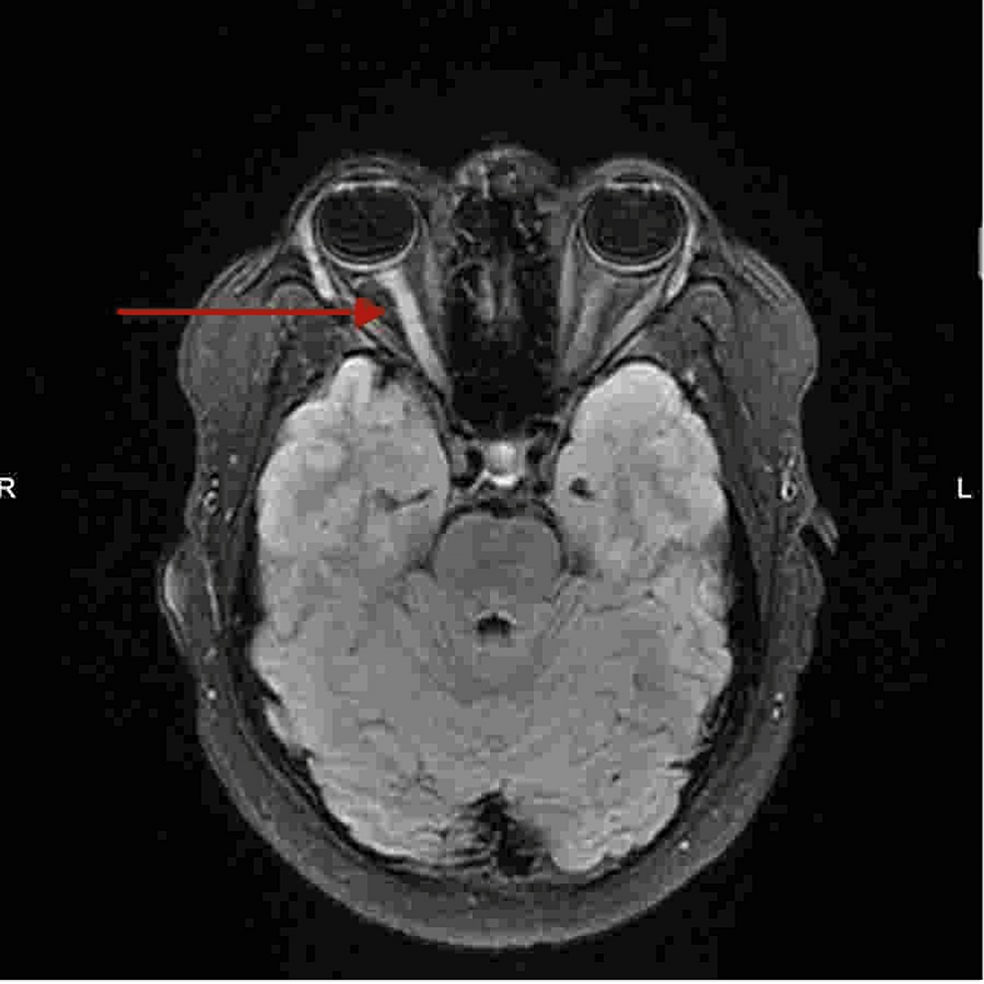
MRI of the brain and orbit T2/FLAIR signal of the aforementioned segments of the right optic nerve is also increased compared to the left side with increased contrast enhancement more prominent at the pre-chiasmatic segment (arrow). MRI: magnetic resonance imaging, FLAIR: fluid-attenuated inversion recovery
